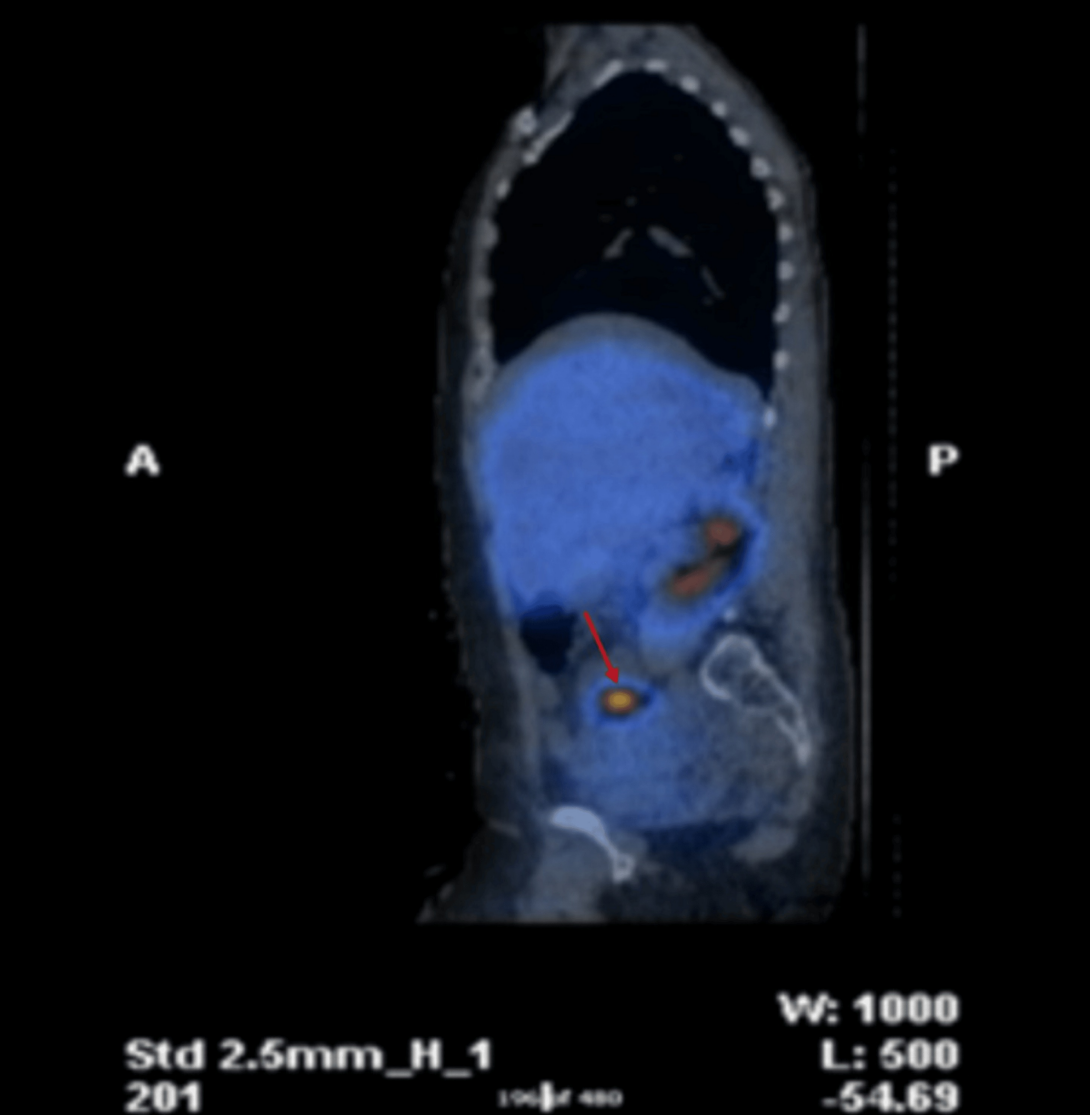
Octreotide scan with single photon emission computed tomography (SPECT) CT showing a 2.5 cm ileal mass (red arrow) with avid uptake of tracer.
